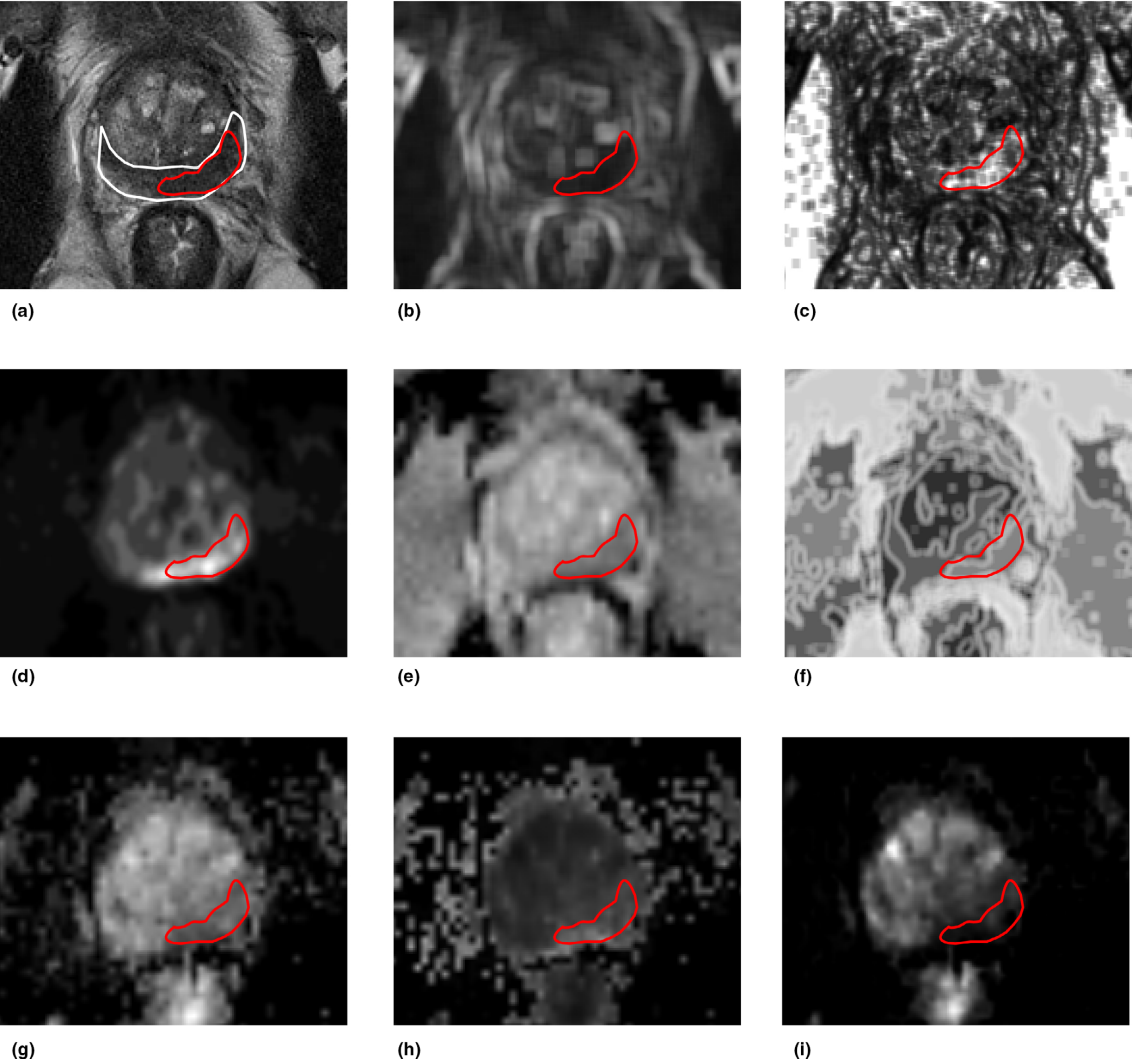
Examples of extracted features from multiparametric magnetic resonance imaging (mp‐MRI) from a 68‐yr‐old prostate cancer patient with serum prostate‐specific antigen (PSA) of 21.0 ng/ml, who was diagnosed with biopsy‐proven Gleason grade 4 + 3 in the left peripheral zone (PZ). Magnetic resonance imaging (a) axial T2WI with delineation of PZ (white) and cancer (red) regions of interest (ROI)s, (b) standard deviation of T2WI, (c) energy map of T2WI, (d) sum average of high b‐value DWI, (e) apparent diffusion coefficient (ADC) map, (f) sum entropy of ADC, (g) λ_3_, (h) attenuation coefficient (AC) of DTI and (i) volume diffusivity of DTI.
